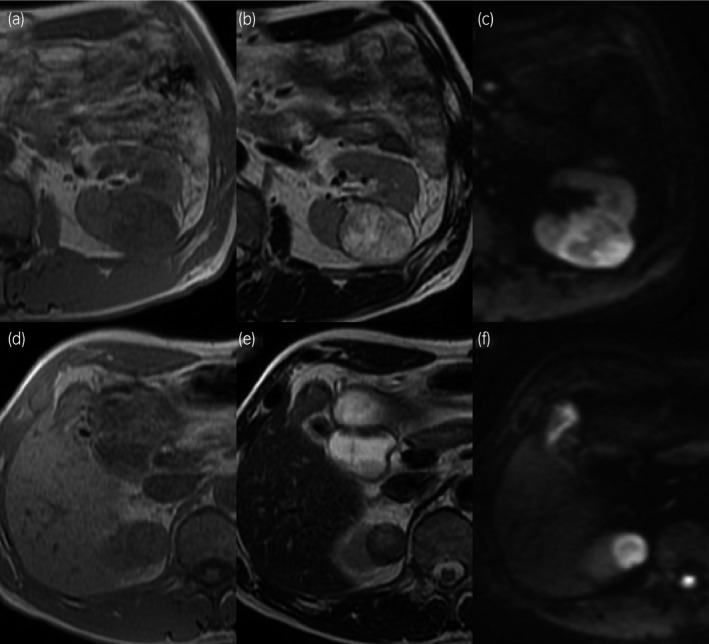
Abdominal MRI of bilateral renal tumors. The left renal tumor shows solid hypointensity on T1‐weighted imaging (a) and irregular hyperintensity on T2‐weighted imaging (b). The right renal tumor appears isointense on T1‐weighted imaging (d) and hypointense on T2‐weighted imaging (e). Both tumors show strong signals on DWI (left; c and right; f).
